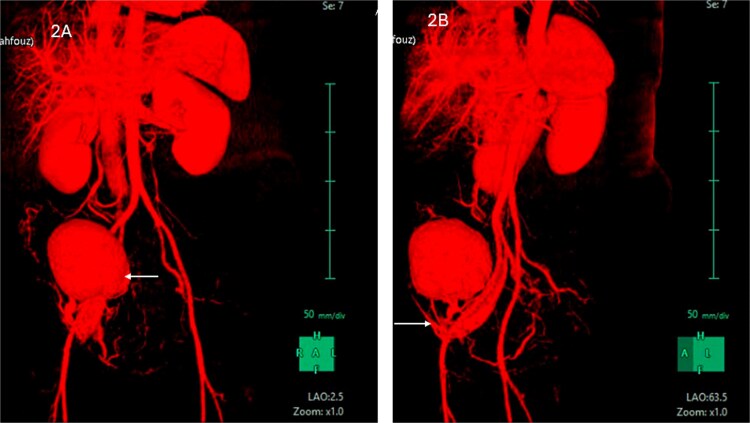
(A) CT abdomen with contrast demonstrating the mass in the right iliac fossa. (B) The image is rotated to show the blood supply from right external iliac artery and vein.
